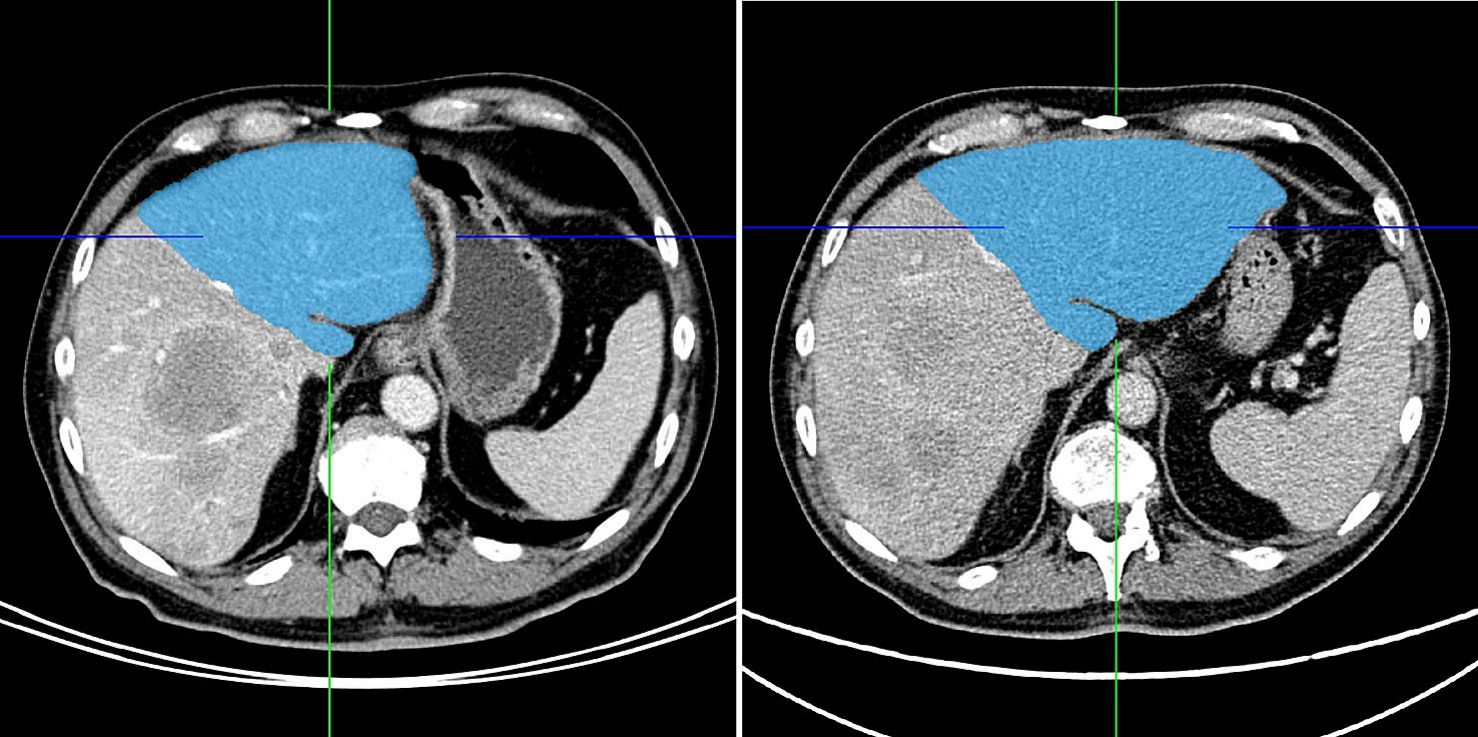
CT scans before (left) and about two months after TARE (right) with volumetry of the contralateral liver lobe (blue)
